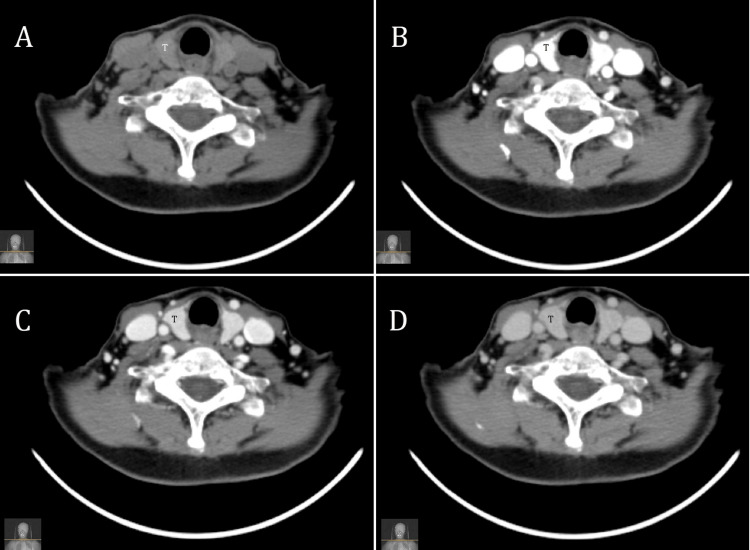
Four-dimensional computed tomography scan of the neck at the level of the thyroid gland. This is an axial CT scan of the neck at the level of the thyroid gland (T) for a 32-year-old female patient in four different phases; Pre-contrast phase (A), arterial phase (B), venous phase (C), and delayed phase (D).
